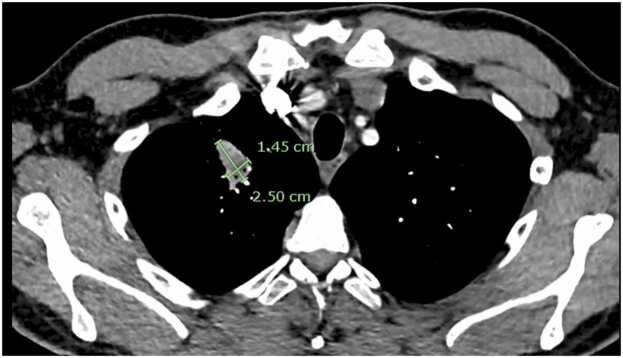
Axial Computed Tomography (CT) of the chest with a 1.45 × 2.5 cm spiculated pulmonary nodule.
